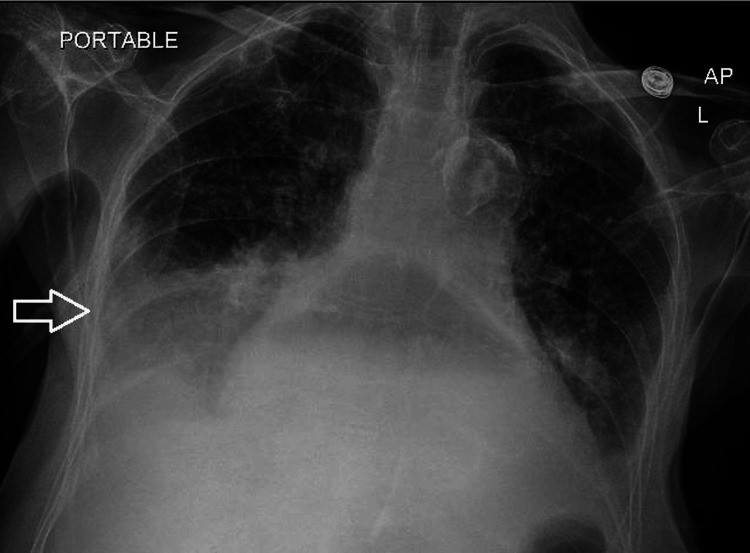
Portable chest radiograph demonstrating right basilar consolidation. Portable anteroposterior (AP) chest radiograph demonstrates right lung base consolidation (arrow), consistent with atelectasis and/or pneumonia. Small bilateral pleural effusions are present. The study also shows cardiomegaly and a large hiatal hernia containing stomach with a visible air-fluid level, consistent with intrathoracic herniation of gastric contents.
